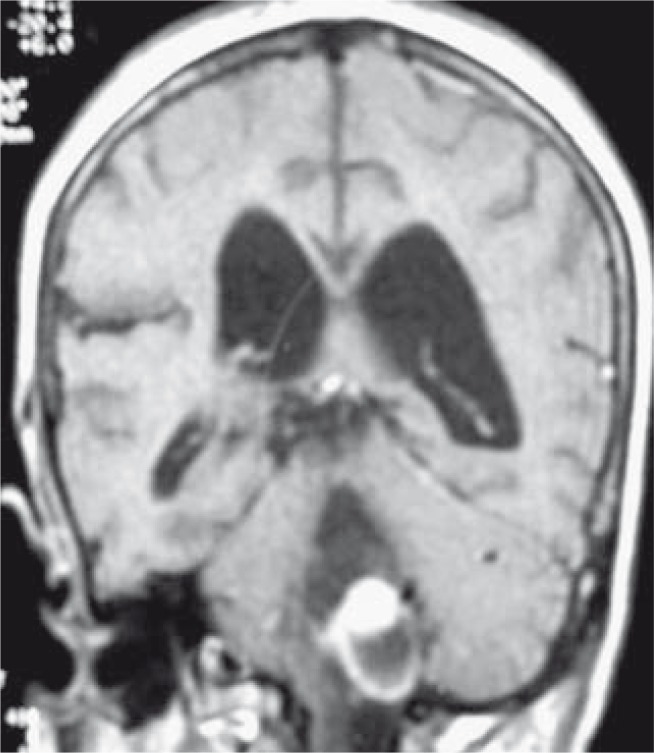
Coronal T1 post-contrast MR image shows a large cystic tumour with enhancing mural nodule in medulla oblongata. There are surgical changes in the *posterior fossa* with dilated fourth and lateral ventricles.
